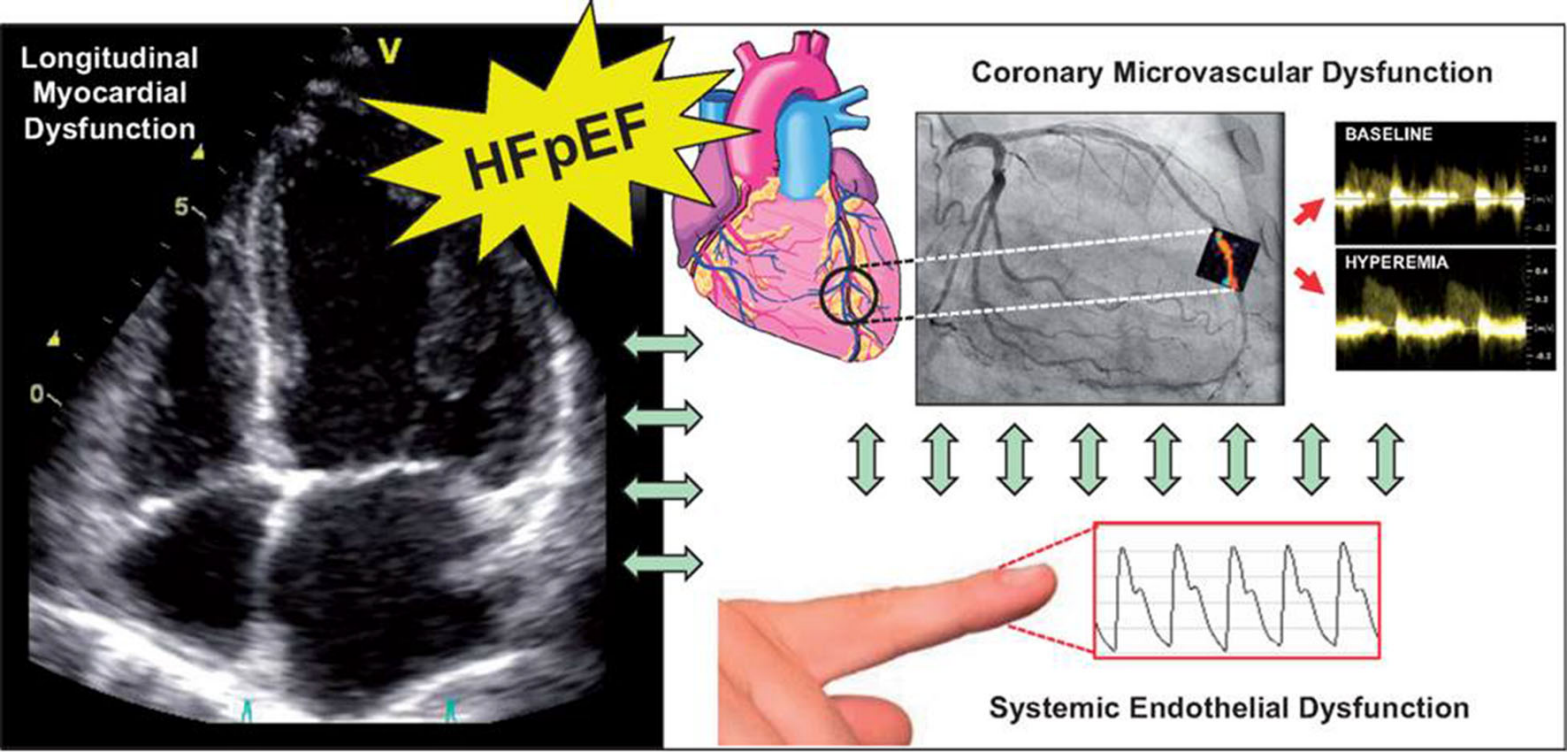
PROMIS-HFpEF study algorithm. In patients diagnosed with heart failure with preserved left ventricular ejection fraction (HFpEF) and nonsignificant coronary artery disease, coronary microvascular dysfunction was assessed based on quantification of coronary flow reserve with adenosine stress transthoracic Doppler echocardiography. In addition, conventional and speckle-tracking echocardiography was used to evaluate left and right ventricular systolic function. Peripheral arterial tonometry was used to measure systemic endothelial dysfunction. Reproduced with permission from Shah et al^1^
